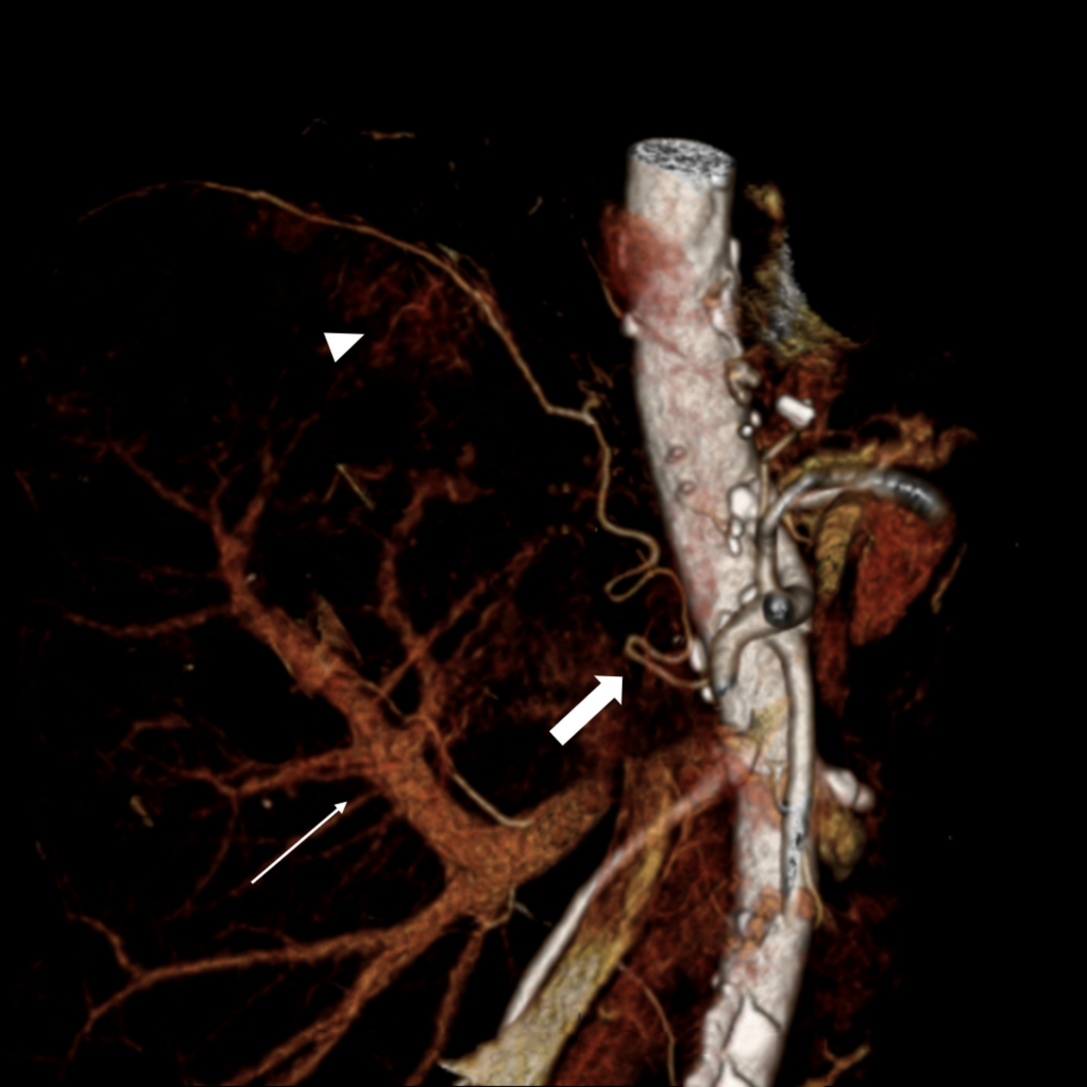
Computed tomography, 3D reconstruction Hypertrophied and parasitized right phrenic artery (thick arrow) providing extra-hepatic collateral supply to the subdiaphragmatic enhancing nodular component within the embolization cavity (arrowhead). Early enhancement of the portal venous system is also seen (thin arrow). 3D: three-dimensional
